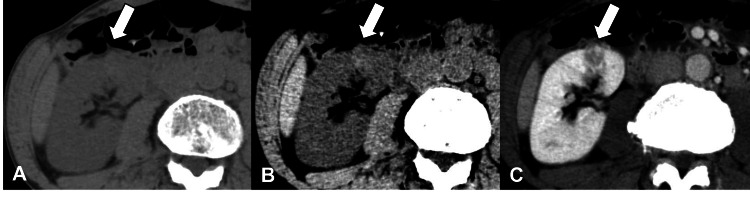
CT images of a 70-year-old man with clear cell RCC A; UE-CT, width: 400 HU, level: 40 HU; B: UE-CT, width: 185 HU, level: 45 HU; C: enhanced image in nephrographic phase On axial UE-CT, a 1.2-cm Hgy low-density mass with unclear margins is detected in the right kidney (arrows). The mass was undetectable on unenhanced scans, but it could be detected by using the ‘‘liver window’’. Pathologic examination confirmed that the mass was a 1.2-cm clear cell RCC RCC: renal cell carcinoma; UE-CT: unenhanced computed tomography
